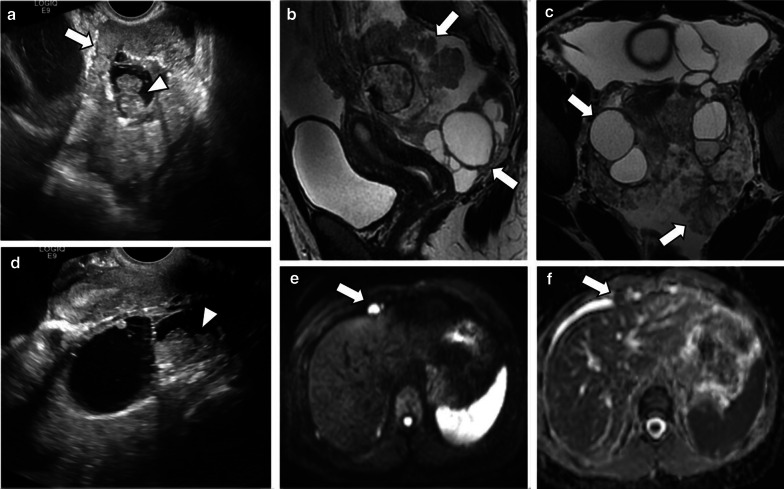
Bilateral LGSC with SBT and peritoneal metastases in a 25-year-old female. Ultrasound images (a, d) reveal a complex mixed tumour with a multinodular aspect involving adnexal structures (arrow). There is fallopian tubes' dilatation with solid echogenic vegetations inside (arrowheads). Sagittal and axial T2-weighted MR images (b, c) demonstrate a bilateral adnexal mixed tumour, with an arborescent growing on the surface of both ovaries (arrows) associated with severe ascites. Axial *b*-1000 s/mm^2^ diffusion-weighted image (e) and ADC map (f) show diffusion restriction in a perihepatic peritoneal metastasis (arrows)
